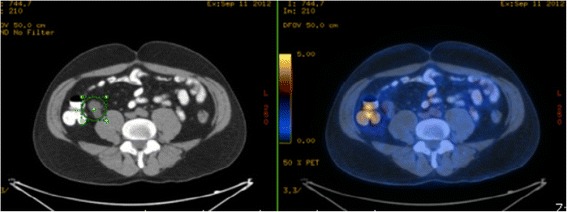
PET-CT of a patient subjected to appendectomy to treat acute appendicitis with incidental diagnosis of appendiceal tubular adenocarcinoma with signet-ring cell formation T3NXMX. In postoperative follow-up, CEA was elevated and the PEC-CT revealed pericecal lymphadenopathy
